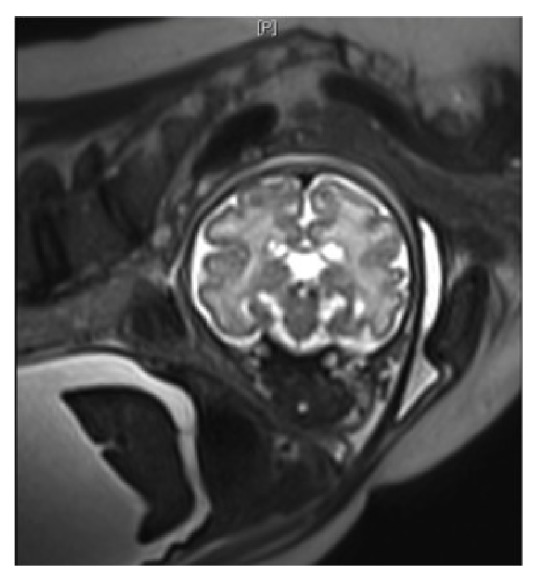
Cephalic antenatal fetal MRI. Coronal T2. Cysts of the caudate nuclei.
